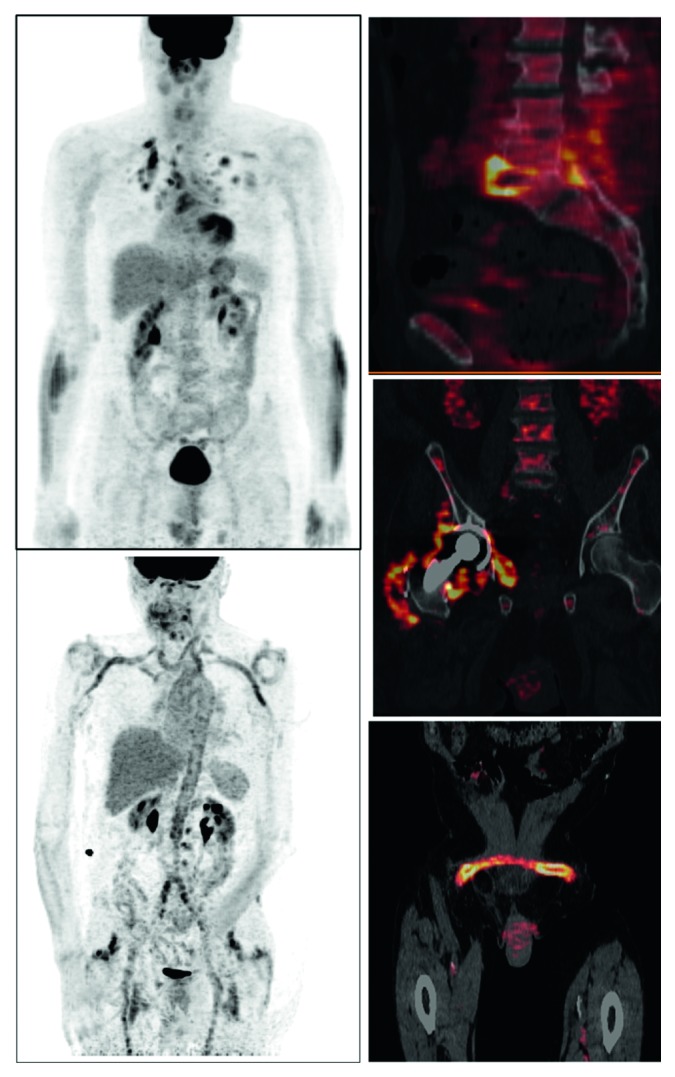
Examples of infectious and inflammatory diseases detected by ^18^F-FDG PET/CT: systemic sarcoidosis with pulmonary and mediastinal involvement (upper left), large vessel vasculitis associated with inflammatory rheumatic disease (lower left), spondylodiscitis (upper right), periprosthetic joint infection (middle right), and vascular graft infection (lower right).
